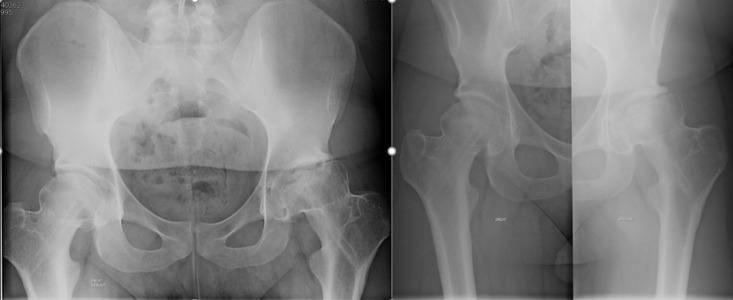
radiographie de contrôle à 9 ans post opératoire initiale, on note la coxarthrose gauche évoluée
